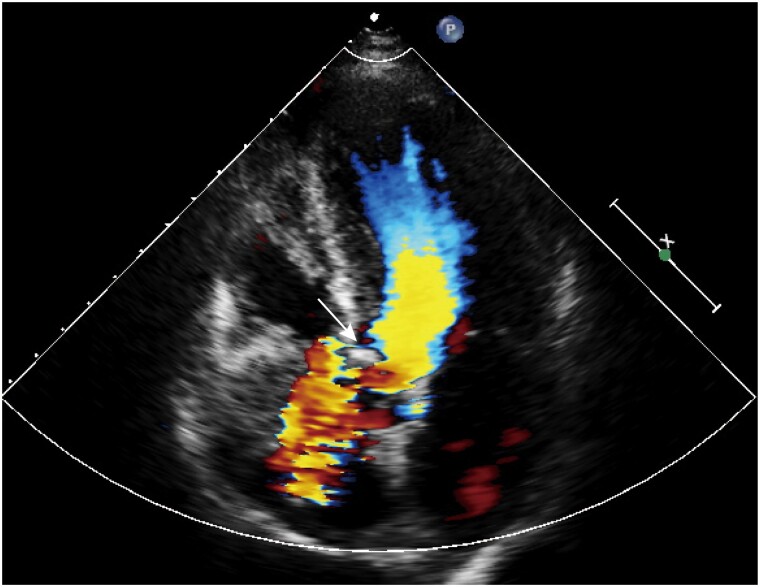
A Doppler flow imaging in the apical five-chamber view of the transthoracic echocardiography. Transthoracic echocardiographic image with colour Doppler flow showing the probable shunt (arrow) between left ventricular and aorta.
